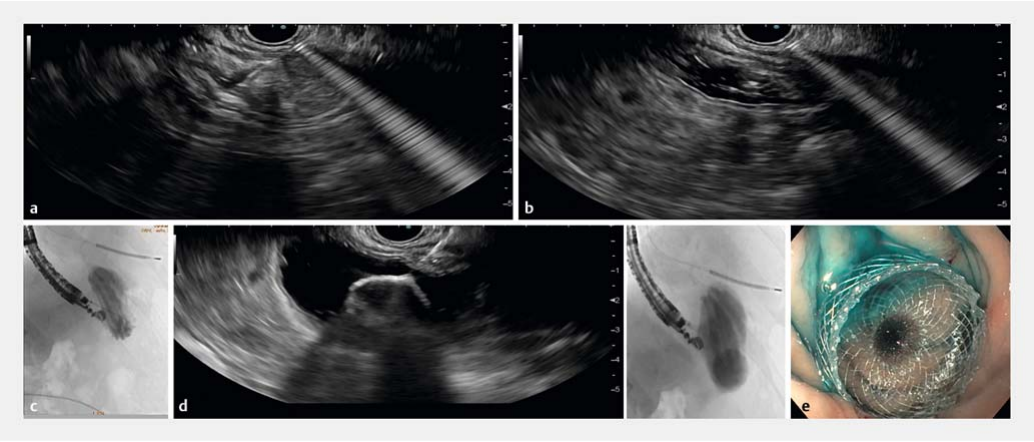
Endoscopic ultrasound (EUS)-guided placement of lumen-apposing metal stent (LAMS).
